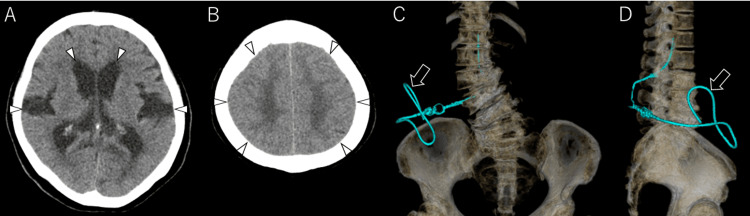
LP shunt for idiopathic normal pressure hydrocephalus (A, B) Preoperative head CT scans reveal ventricular enlargement, widening of the Sylvian fissures, and narrowing of the cortical sulci in the convexity (arrowhead). (C: frontal view, D: right lateral view) Postoperative 3D CT scans demonstrate the distal catheter inserted into the right abdominal cavity following the placement of the LP shunt (arrow).
